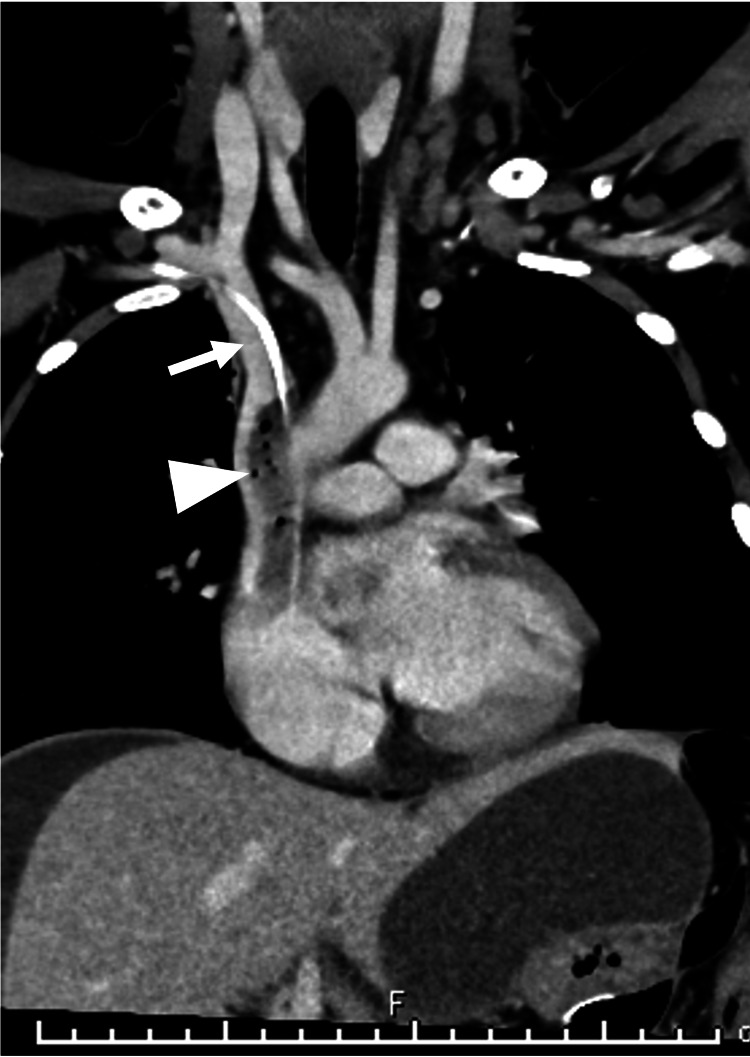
Contrast-enhanced computed tomography on the same day as the first Thrombosis (arrowhead) connected with the tip of the peripherally inserted central catheter (white arrow).
